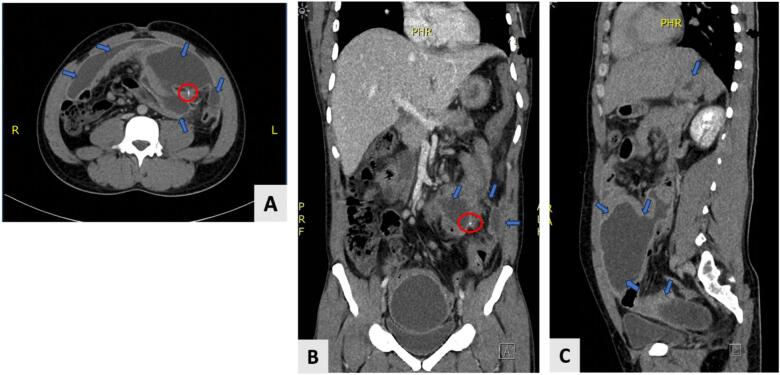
CT scan of the abdominopelvic region. A: Axial view. B: Coronal view. C: Sagittal view. Blue arrows pointing toward multiple sites of abscesses. Red circle encircles a cross section of the foreign body. (For interpretation of the references to colour in this figure legend, the reader is referred to the web version of this article.)
